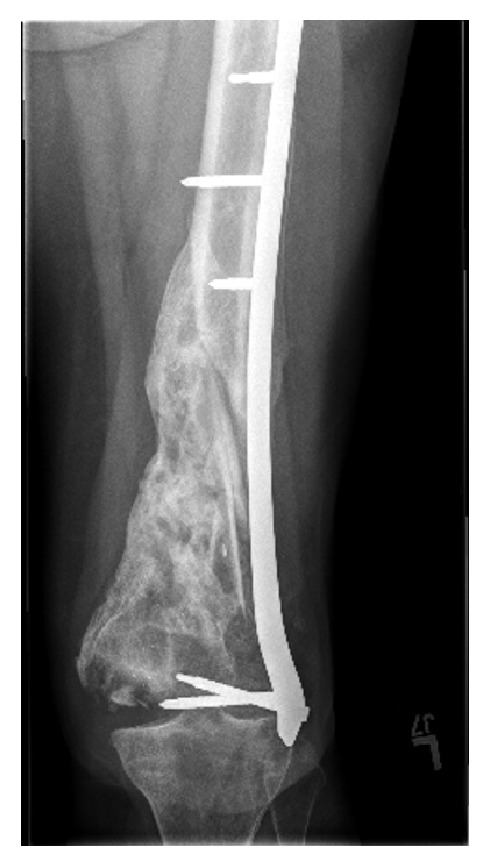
AP radiograph of distal femur.
